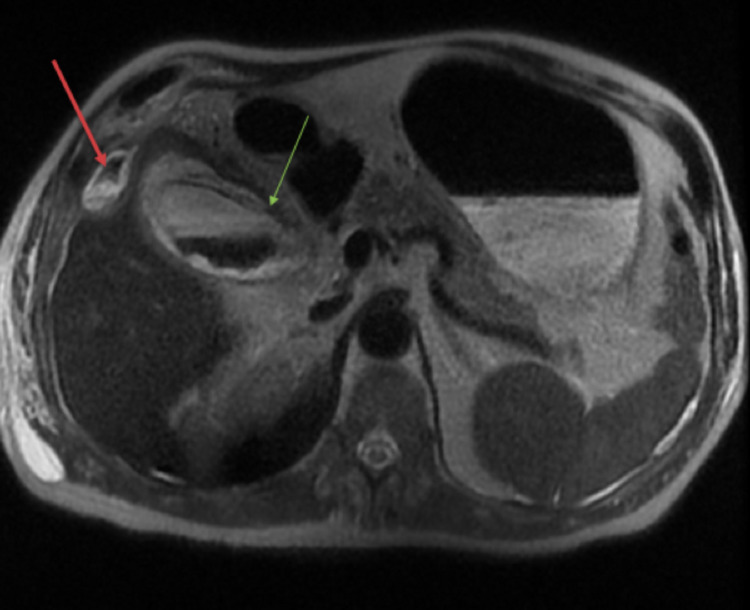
Magnetic resonance (MR) T2-weighted (axial view) findings consistent with perforated gangrenous cholecystitis. Note there is fundal wall discontinuity (green arrow) and subjacent right upper quadrant and subcapsular abscesses/phlegmonous change (red arrow).
